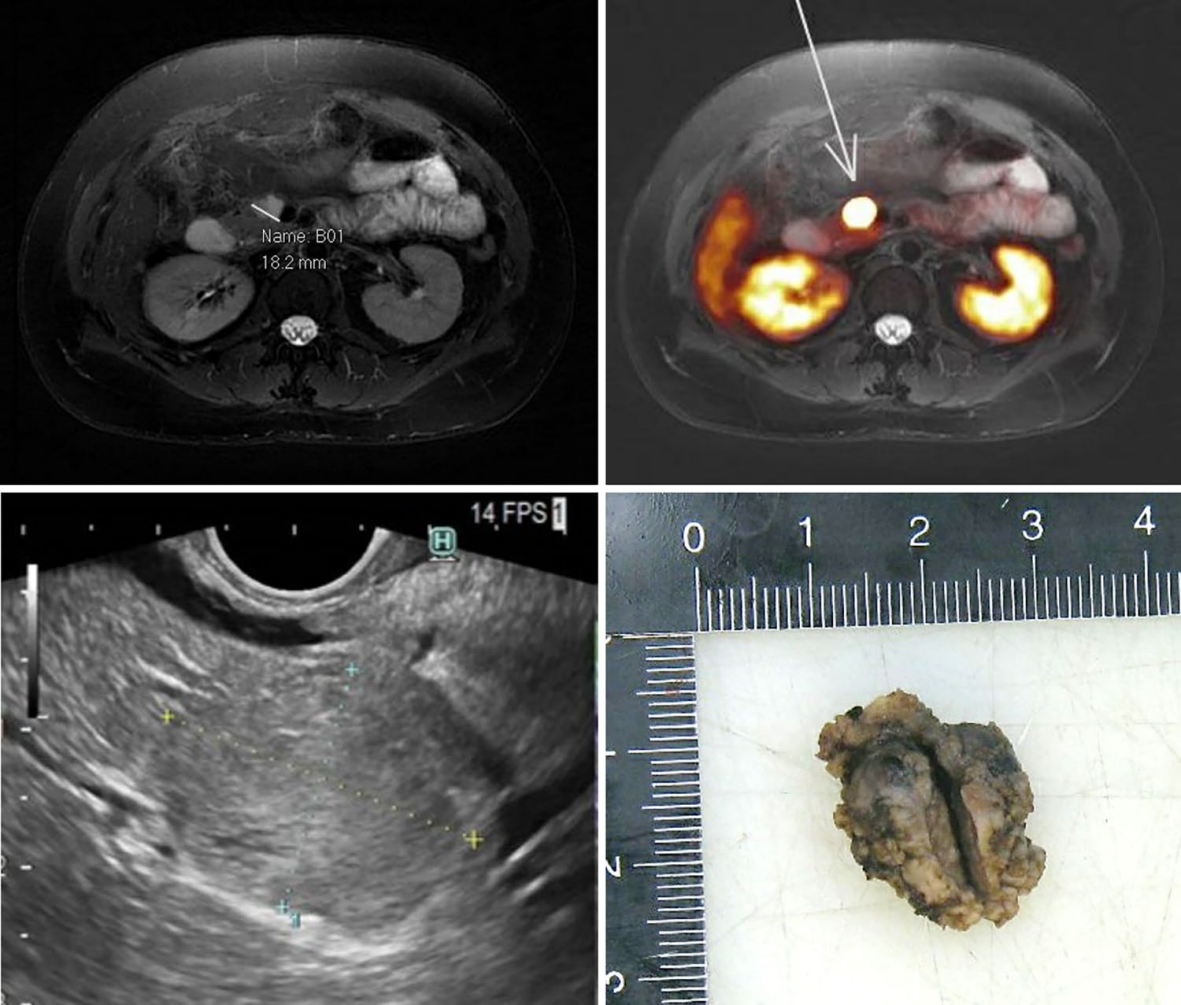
43-year-old woman with hyperinsulinemic hypoglycemia caused by an SSTR2-positive Grade 1 T1 insulin-producing pancreatic NET with a diameter of 1 cm. Upper panels, from left to right: MRI (T2W) shows a high T2 intense hypervascular lesion in the region of the pancreatic head which is positive on the ^68^Ga-DOTATATE PET MRI. Lower panels, from left to right: endoscopic ultrasound shows an isoechoic lesion that was resected endoscopically
